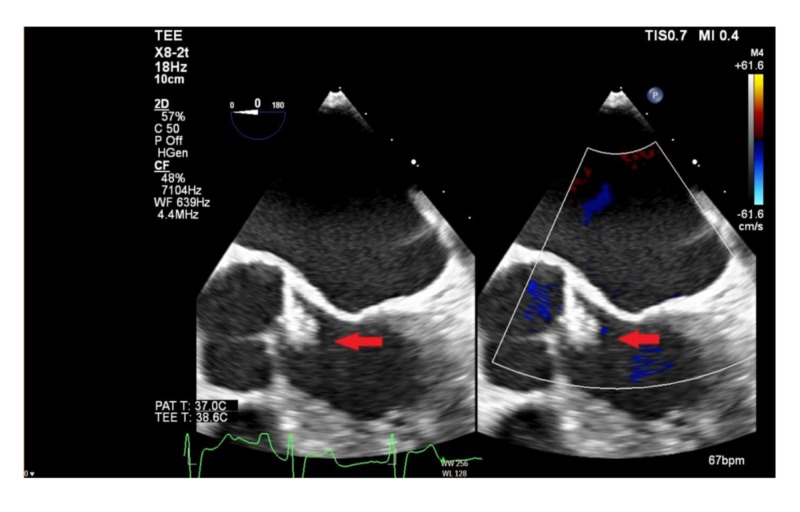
TTE/TEE images of CPFE (red arrow) of the LCC Left picture images without color flow adjustments; right with color flow adjustments TTE = Transthoracic Echocardiography; TEE = Transesophageal Echocardiography; CPFE = Cardiac Papillary Fibroelastoma; LCC = Left Coronary Cusp
